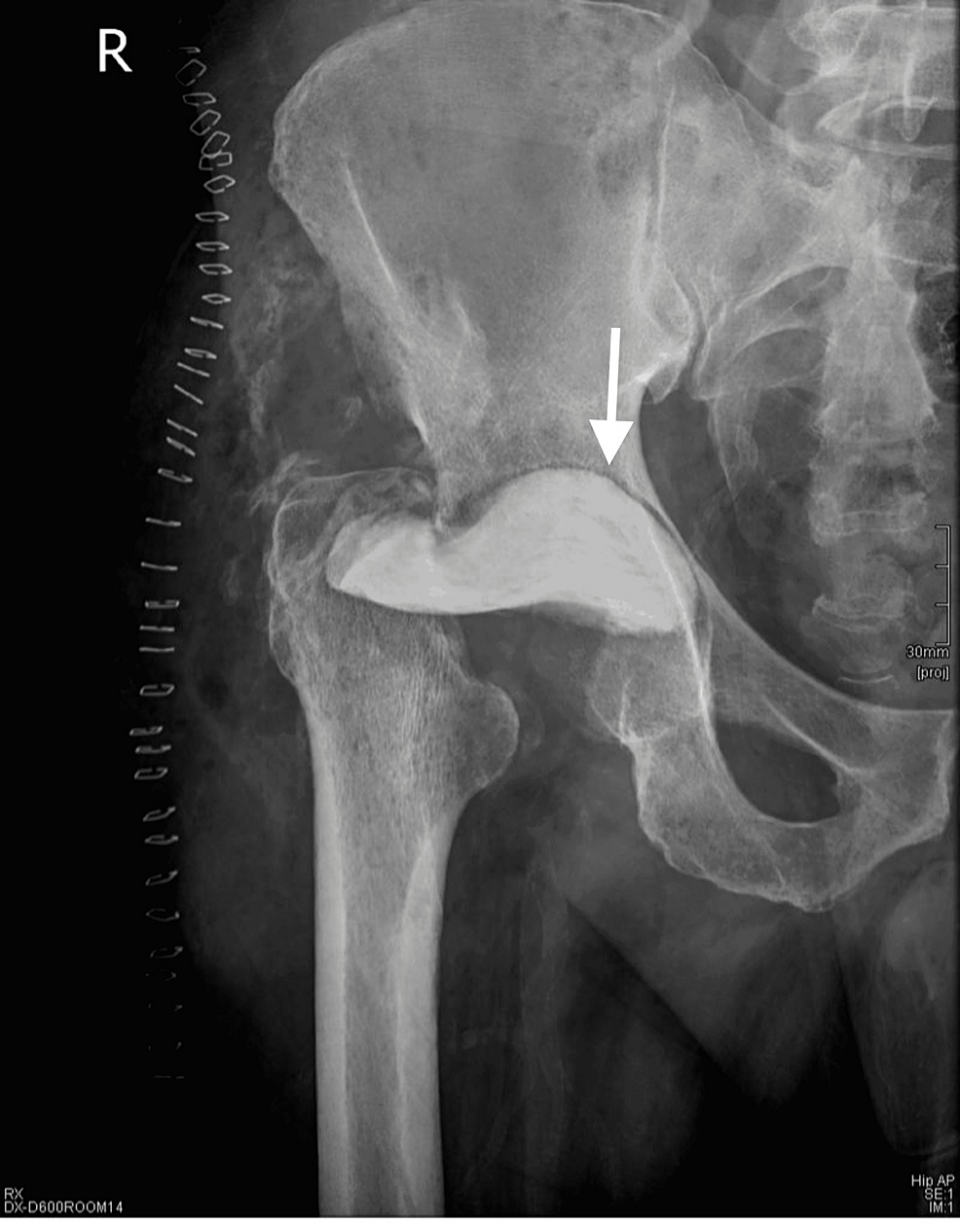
Right hip x-ray after the insertion of the second antibiotic cement spacer.
